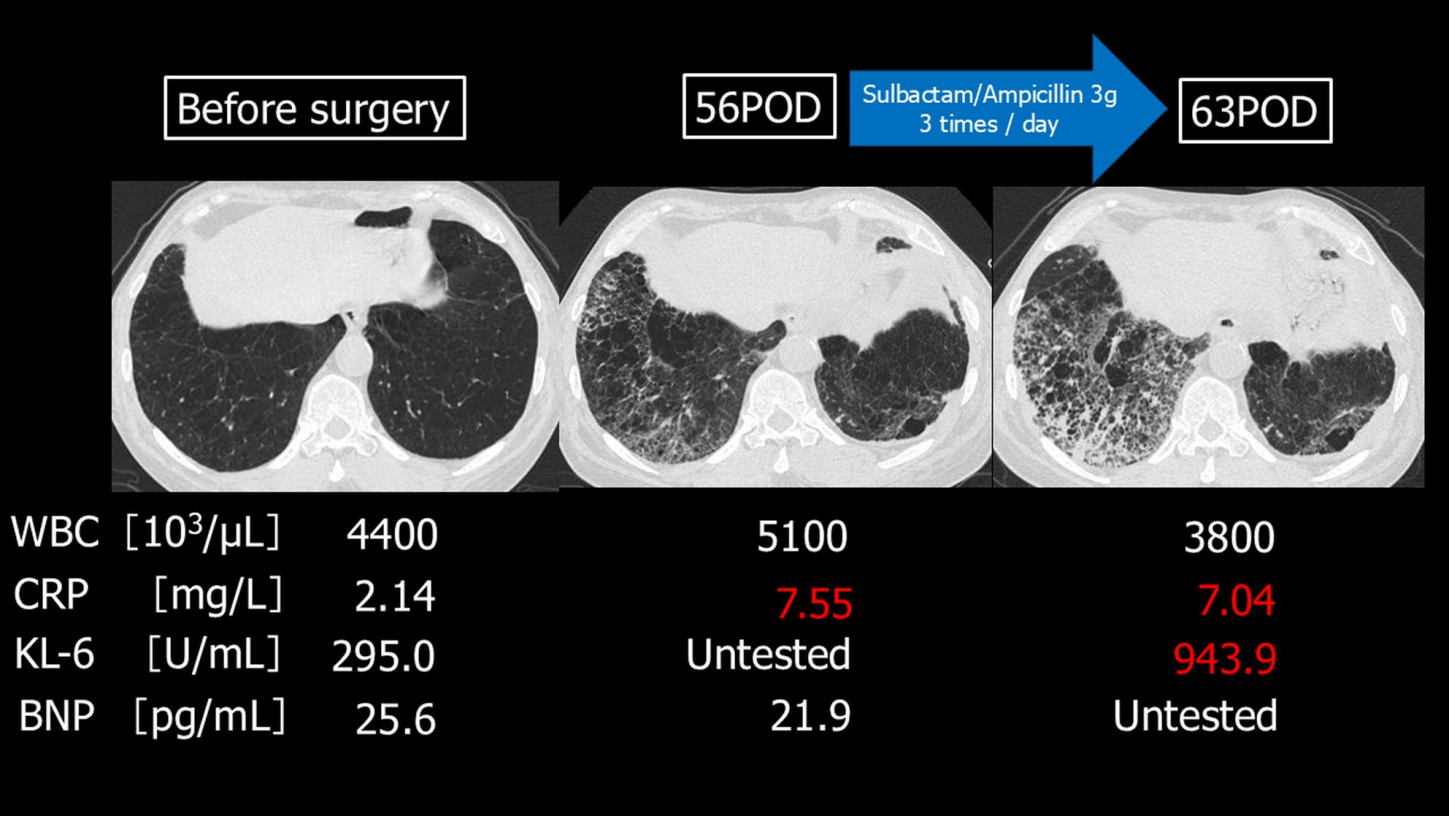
Preoperative and postoperative computed tomography imaging and biochemical test results This image shows the progression of the lower lobe pneumonia on chest computed tomography images before and after surgery, along with the corresponding biochemical test results. On POD 56, pneumonia in the right lower lobe was observed, and the C-reactive protein level was elevated. By POD 63, the pneumonia in the right lower lobe had worsened. POD: Postoperative day
